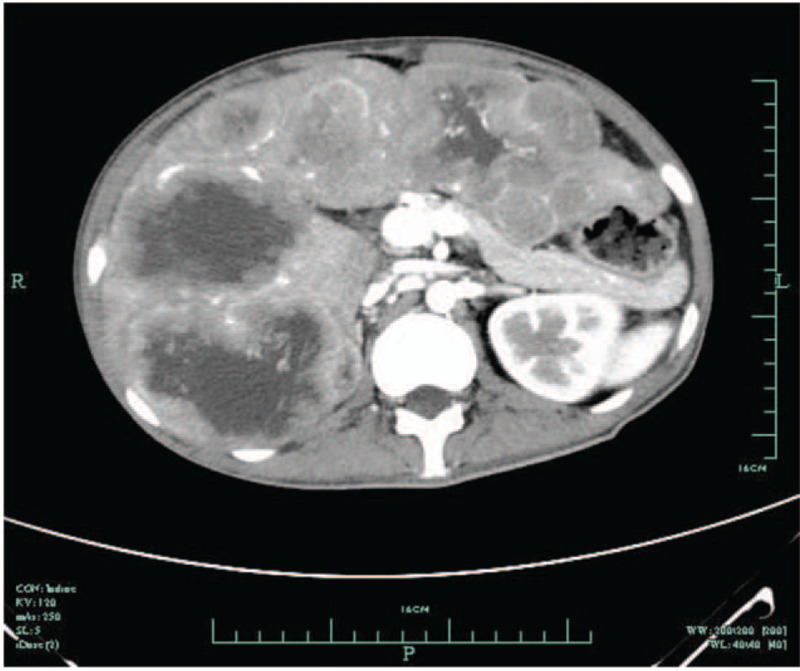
Abdominal computed tomography image. There were multiple sizes of intrahepatic abscesses, and enlarged lymph nodes around the mesenterium and posterior peritoneum as well as ascites.
